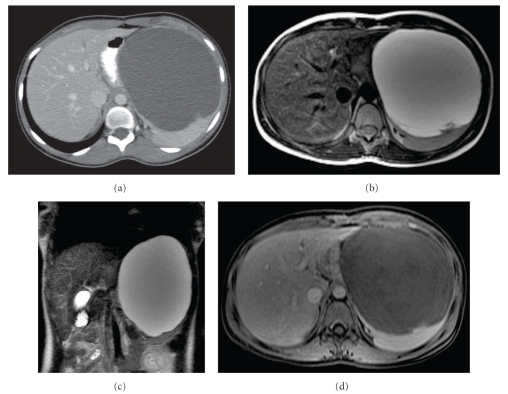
(a)–(d) Preoperative abdominal CT, (a) axial (b) and coronal (c) T2 weighted MRI shows a giant rounded cystic mass anterior to the spleen, which is significantly displaced posteriorly. Axial T1 weighted fat saturated MRI postgadolinium administration shows a thin peripheral enhancement of the splenic mass—possibly representing a capsule—and no internal enhancement consistent with its cystic nature (d).
